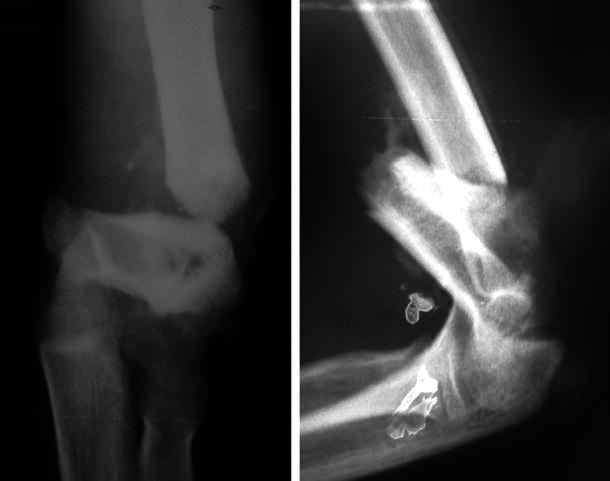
X-ray of a patient with displaced lower fourth fracture humerus neglected for 34 days showing off-ending of the fragments with visible callus formation in the lateral view (patient 8)
